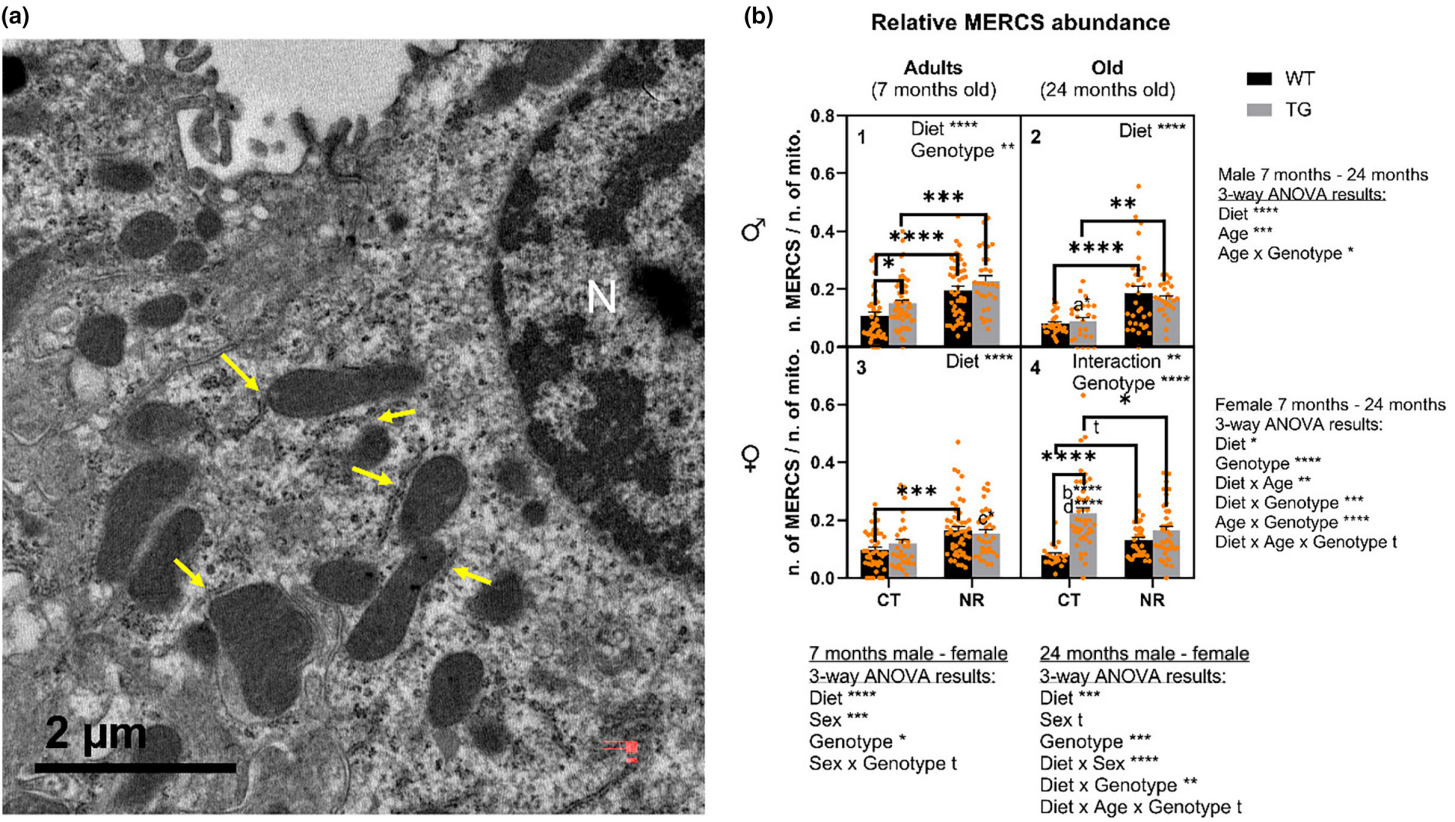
Mitochondria‐endoplasmic reticulum contact sites (MERCS) abundance in distal convoluted tubule cells under the different experimental conditions. (a) Shows some examples of MERCS marked with yellow arrows. (b) Shows relative MERCS abundance. MERCS abundance data are expressed as the number of MERCS per mitochondria (see Materials and Methods). See Figure 5 caption for a description of the panel arrangement. In (b); t, tendency (*p* < 0.1); p* < 0.05; *p* < 0.01; p* < 0.001; *p* < 0.0001; a*, *p* < 0.05 versus AM TG CT; b, *p* < 0.0001 versus AF TG CT; c*, *p* < 0.05 versus AM TG NR; d, *p* < 0.0001 versus OM TG CT.
